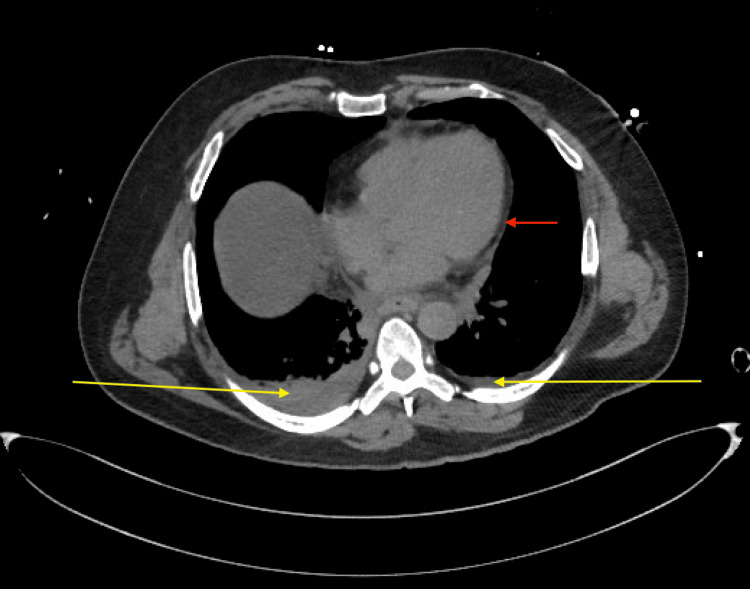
CT chest axial view The scan revealed bilateral mild pleural effusion, more pronounced on the right side (yellow arrows). A mild pericardial effusion was observed (the red arrow) CT: computed tomography
